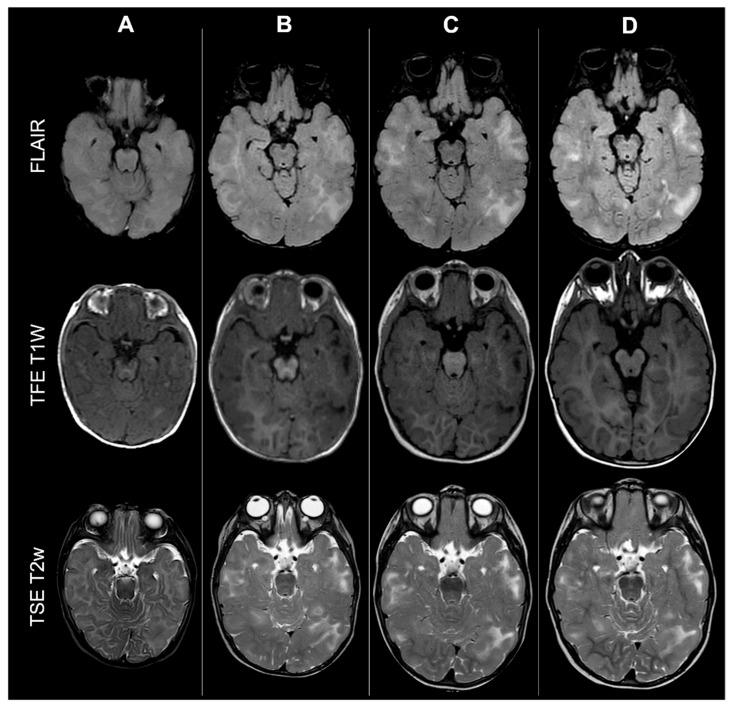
Effect of myelination on cerebral cortical tubers’ count: MRI scans from the same male TSC2 patient acquired at four different timepoints (respectively: 6 months old—column A; 18 months old—column B; 36 months old—column C; 4 years old—column D, showing how incomplete myelination in early infancy may impact tubers detection by reducing contrast with surrounding brain tissue.
